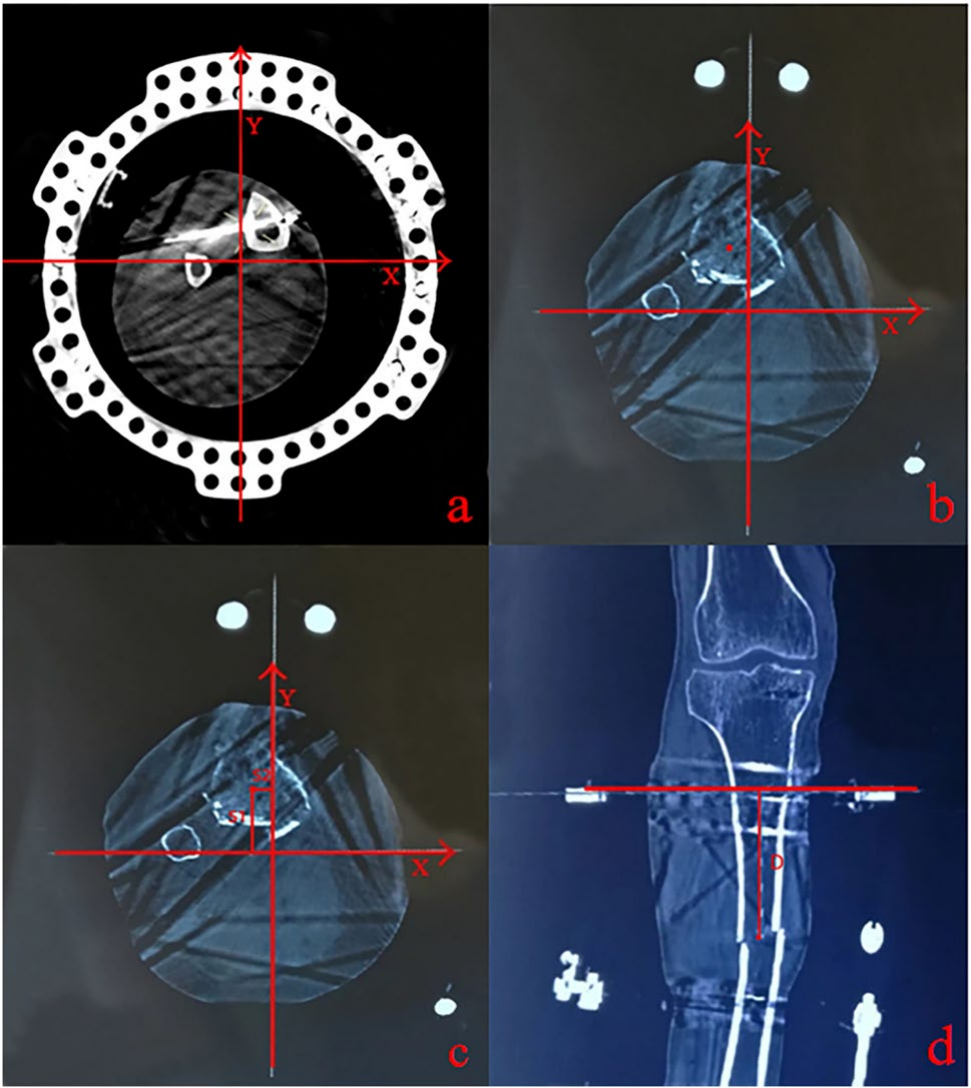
CT measurement of hexapod external fixator mounting parameters. a On the slice where the proximal reference ring is fully visualized in the CT workstation, its center was identified. Spatial coordinate axes for the AP and mediolateral directions were then established across all planes. b The image was navigated to the level of the fracture line, and the midpoint of the diaphysis (red dot) was located. c The distances from the diaphyseal midpoint to the X and Y axes (S1 and S2) were measured, representing the AP and lateral mounting parameters, respectively. d On the coronal image, the vertical distance (D) between the fracture line and the reference ring was measured, which represents the axial mounting parameter
